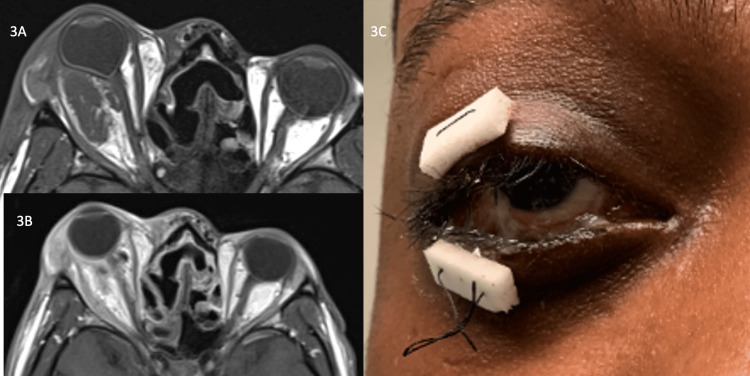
Hospital stay - 3A. MRI orbits axial view demonstrating intraconal abscess with tenting of the globe and straightening of the optic nerve; 3B. MRI following treatment; 3C. Photograph of the right eye following treatment showing improvement in proptosis and chemosis.
